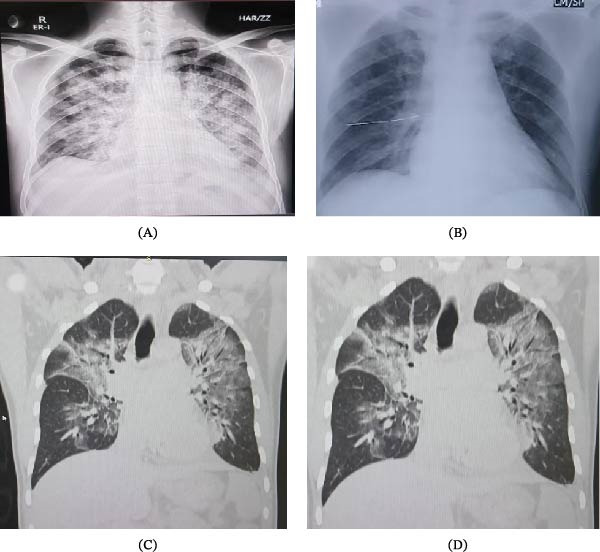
(A–D) Imaging findings of pulmonary congestion/edema. (A) Initial chest X‐ray demonstrating bilateral patchy perihilar and basal opacities, consistent with pulmonary congestion or edema. (B) Follow‐up chest X‐ray showing significant resolution of prior pulmonary congestion after intervention. (C, D) HRCT chest images showing diffuse ground‐glass opacities with interlobular septal thickening in bilateral lungs, consistent with pulmonary edema; no significant consolidation or cavitation is noted.
